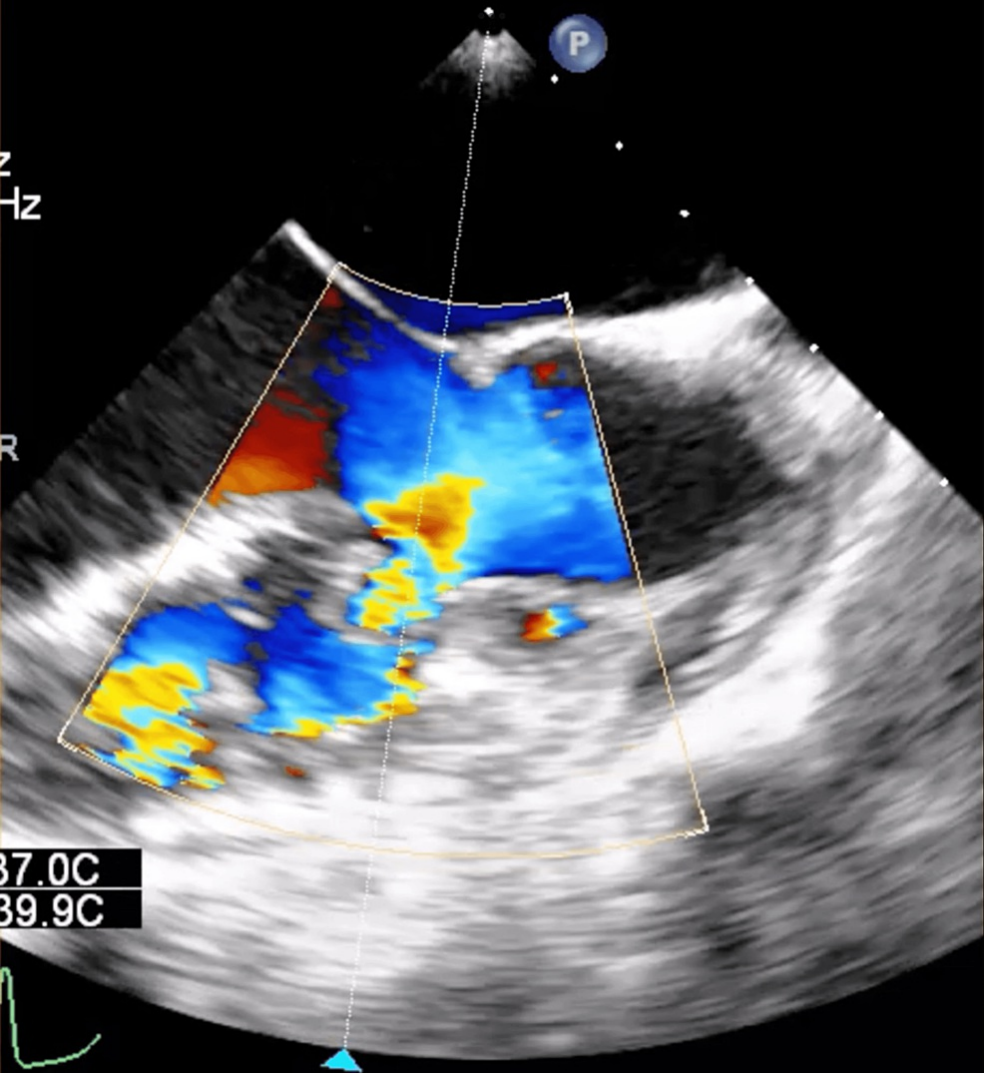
Color Doppler mid-transesophageal echocardiography showing a ventricular septal defect (VSD) from the left to right ventricular side
